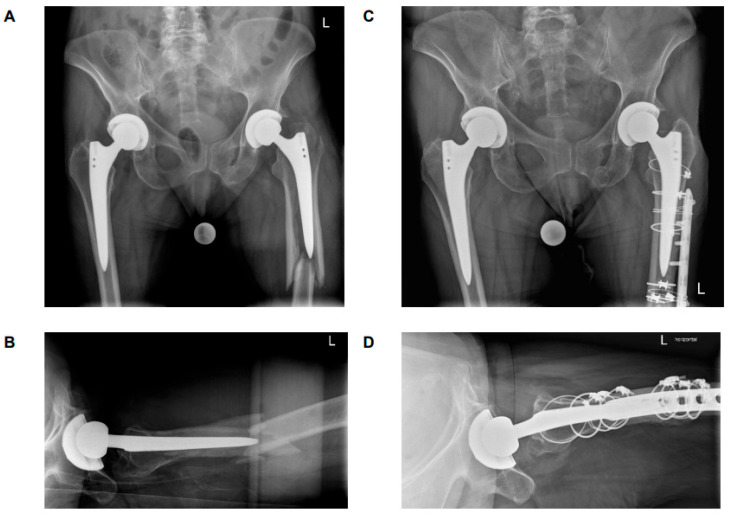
Antero-posterior and axial X-rays. (A,B) preoperatively; (C,D) 52-week-follow-up. A 71-year-old women with PPF, left, ORIF, excellent functional outcome. By definition, the stem is loose, but as the greater trochanter is still attached to the prosthesis, the patient has no limb and no stem subsidence.
